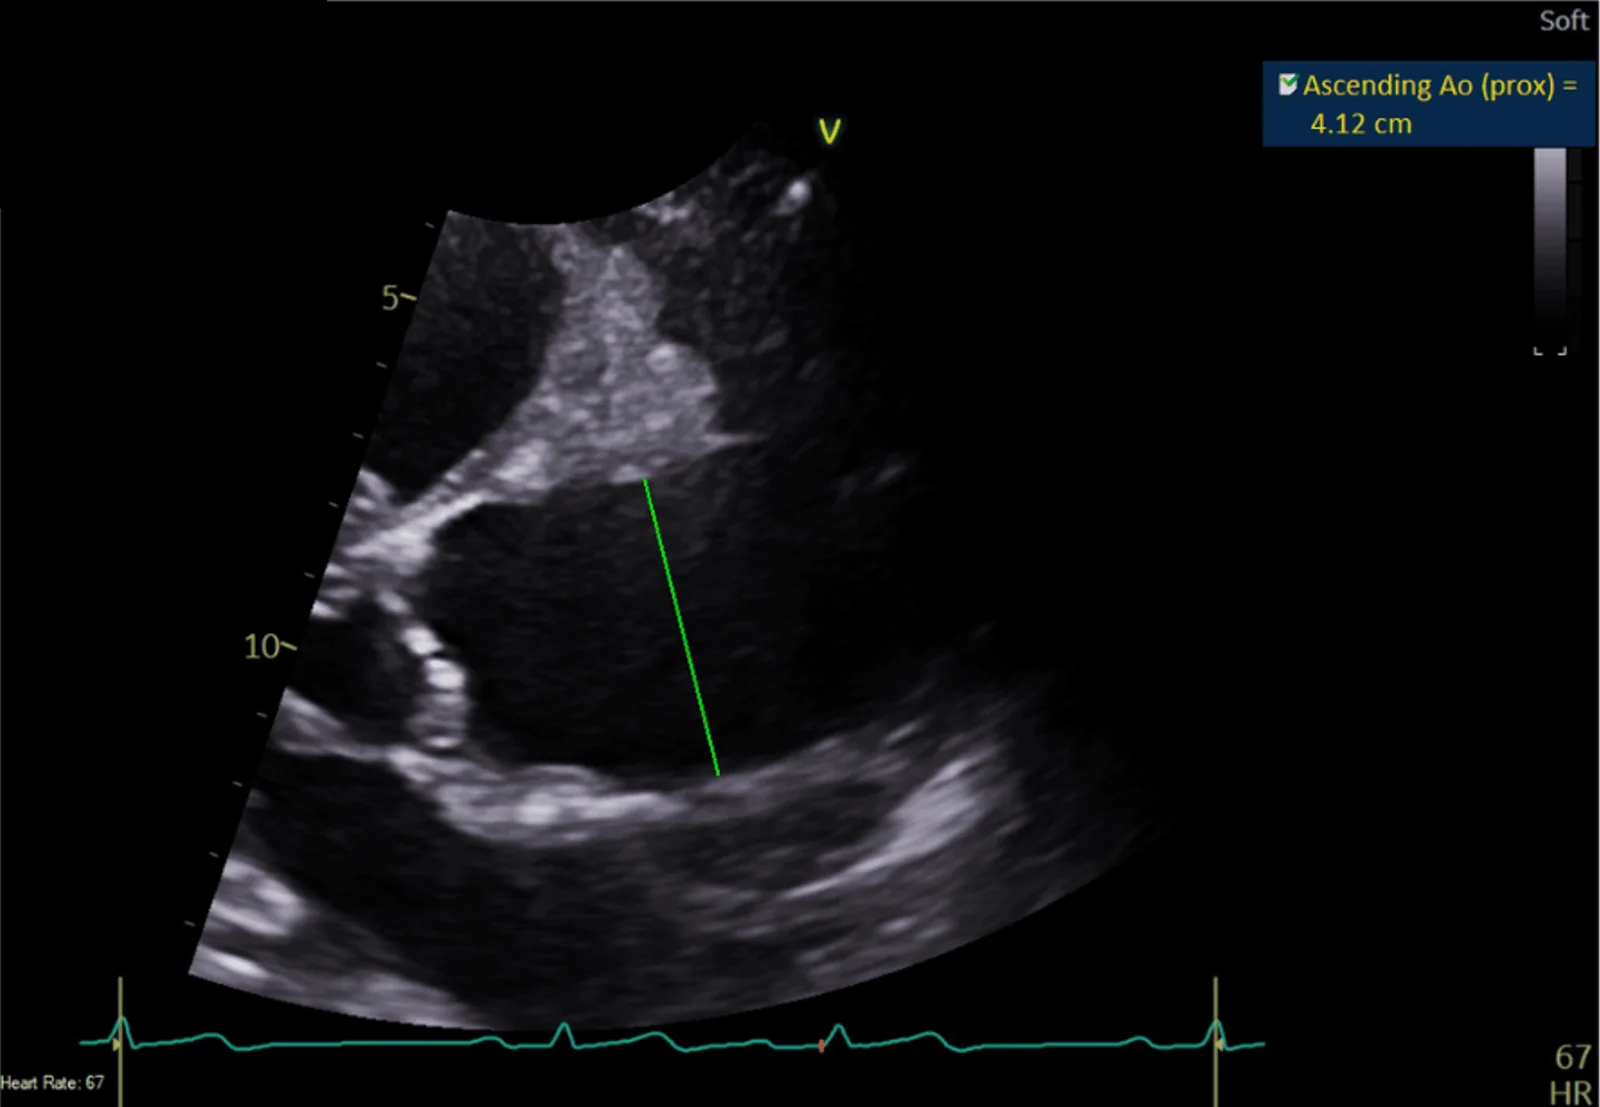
Transthoracic echocardiography (TTE) image showing dilated proximal ascending aorta.
